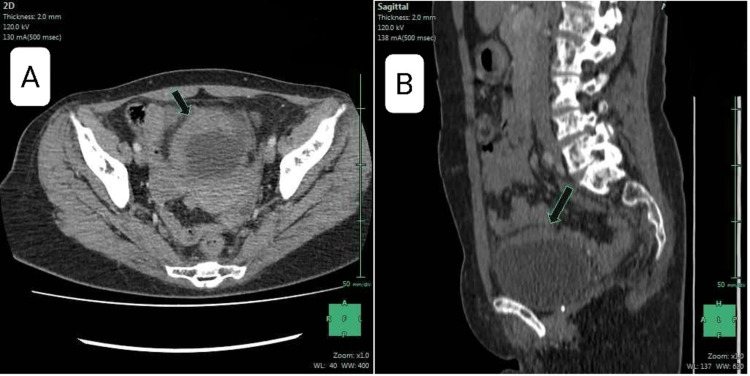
Axial (A) and sagittal (B) contrast-enhanced CT images of the abdomen and pelvis, showing a bladder wall thickening.
